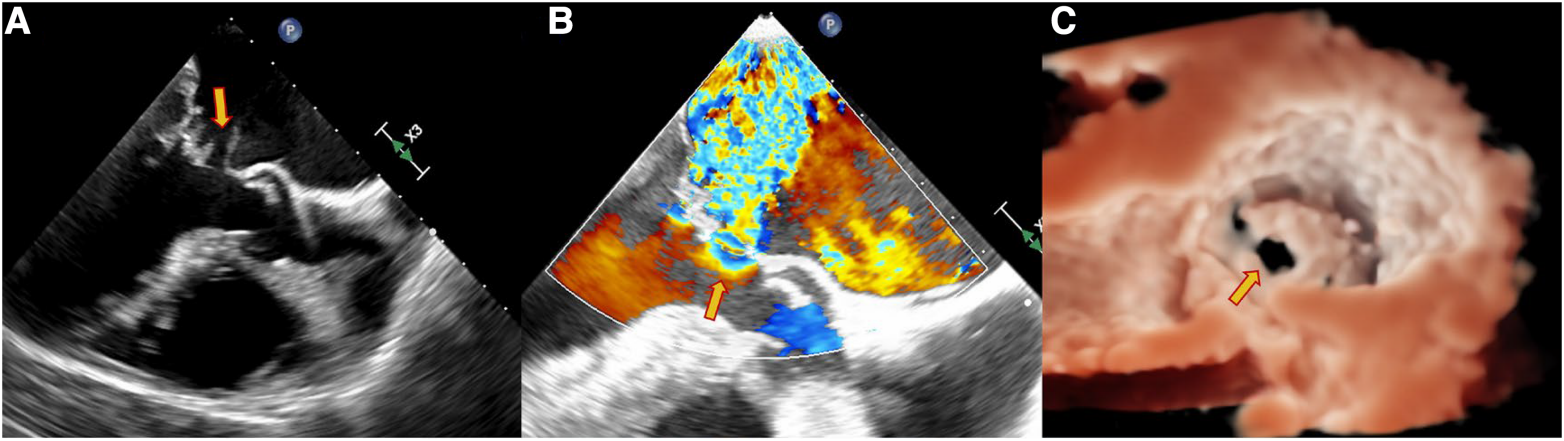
Echocardiography data of a 63-year-old male patient with dyspnea for 4 days. TEE showed the perforation of the MVA as pointed out by the yellow arrow. (A) Continuous interruption of the MVA wall. (B) Regurgitation from the MVA. (C) Continuous breaks on the MVA from a 3D view.
